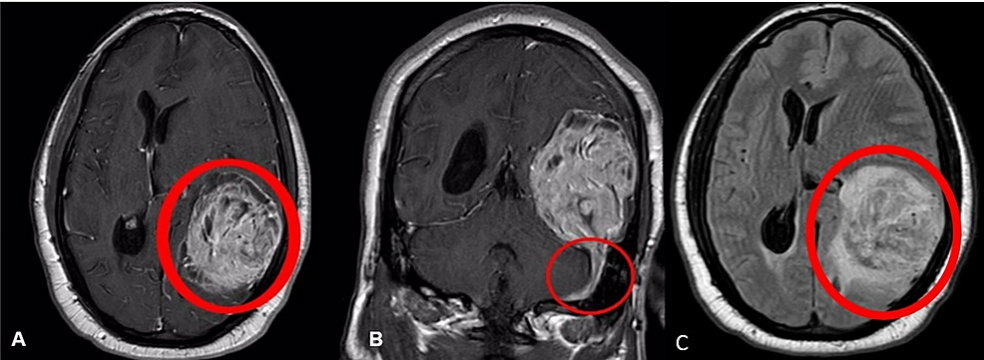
Magnetic resonance imaging (MRI) of the brain (T1 axial sequence with contrast) showing (A) an extra-axial, heterogeneous, highly vascularized 7.6-cm mass compressing the left parietal lobe along with a centimeter left-to-right midline shift (encircled). (B) MRI brain T1 coronal sequence with contrast demonstrating the dural tail (encircled). (C) MRI T2-fluid attenuated inversion recovery (FLAIR) sequence showing an alternating pattern of hypo-intensity and hyperintensity ("yin-yang" sign) along with edema.
